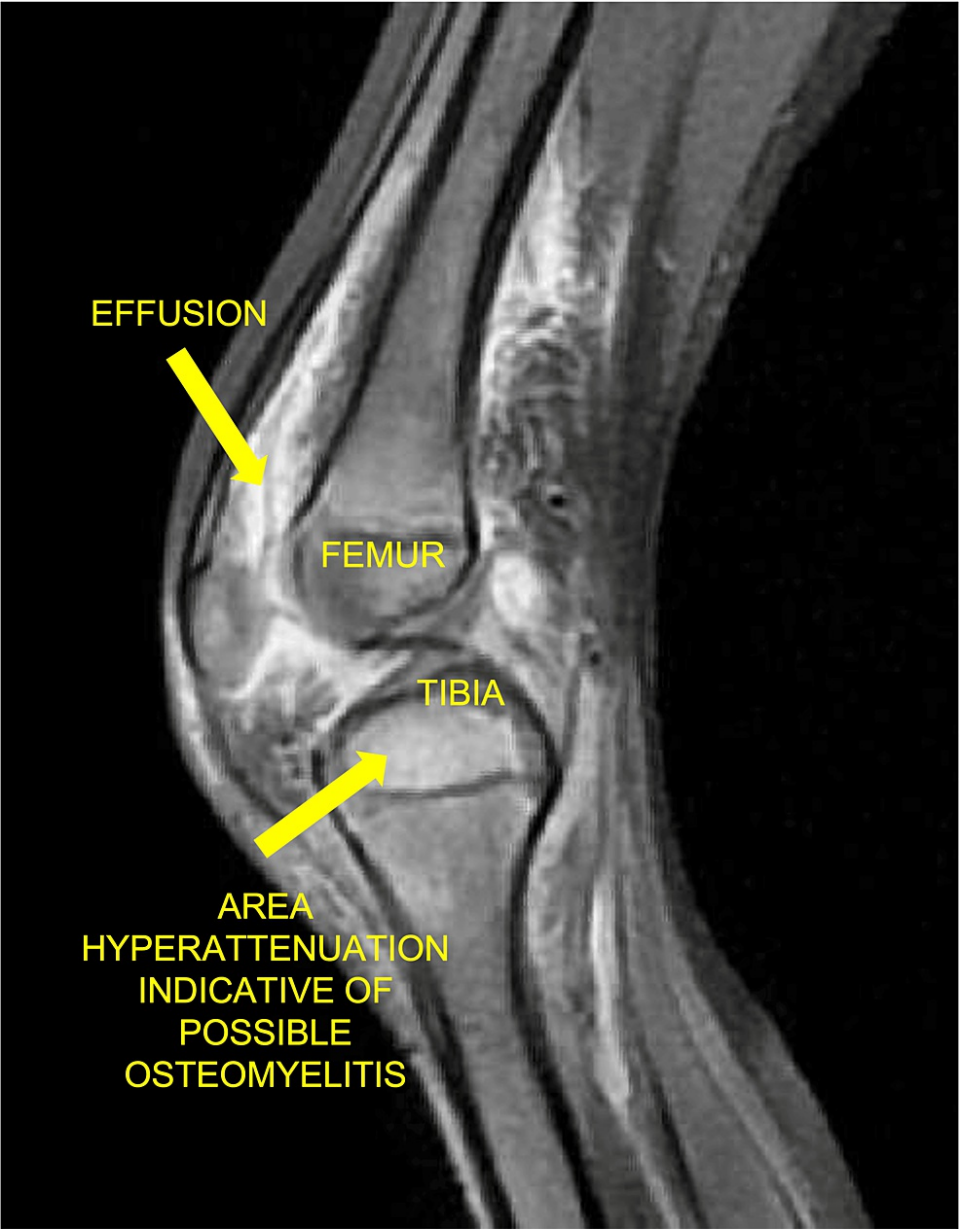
MRI knee of a child with septic arthritis Credit: Image adapted from Radiopaedia [24]. Published under Creative Common License.
